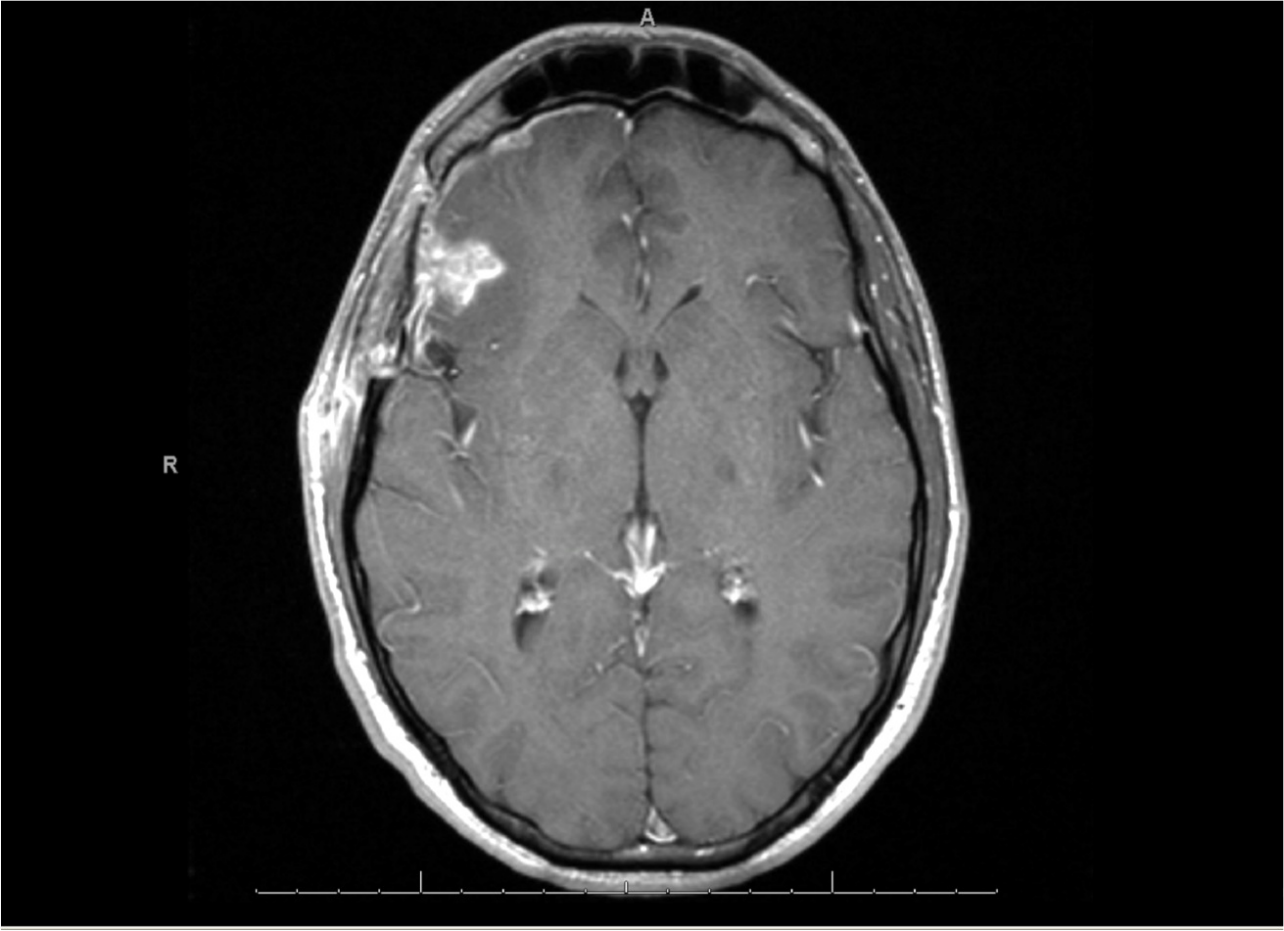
MRI One Month Post-Resection Increased enhancement in the surgical bed.
